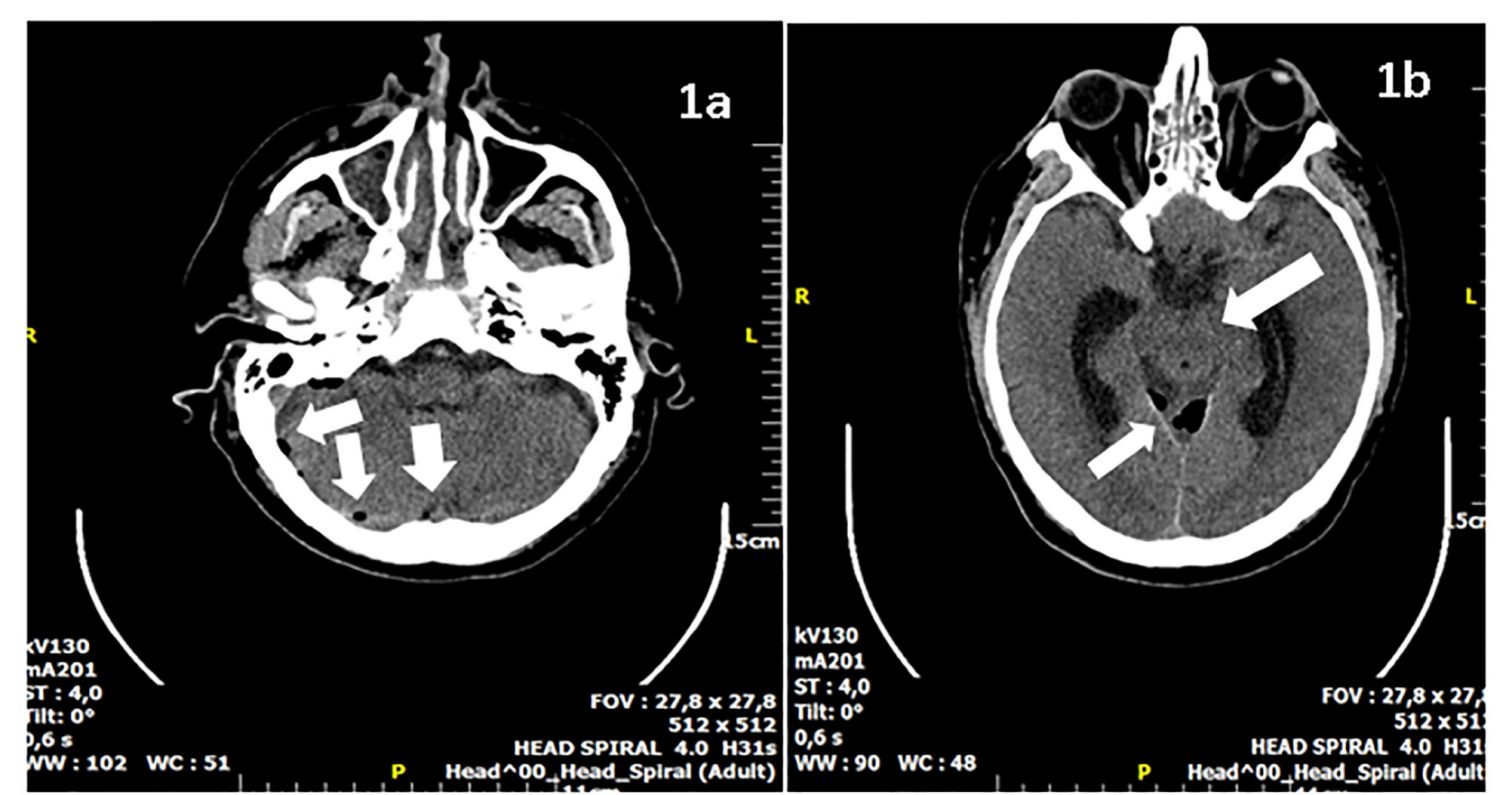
Axial CT brain 1A) Axial brain CT image showing pneumocephalus in the posterior cranial fossa; 1B) Axial brain CT image showing pneumocephalus at the level of the cerebellum in close relation to the right temporal and occipital bones. Diffuse edema of the brain stem and temporal lobes. CT: computed tomography
